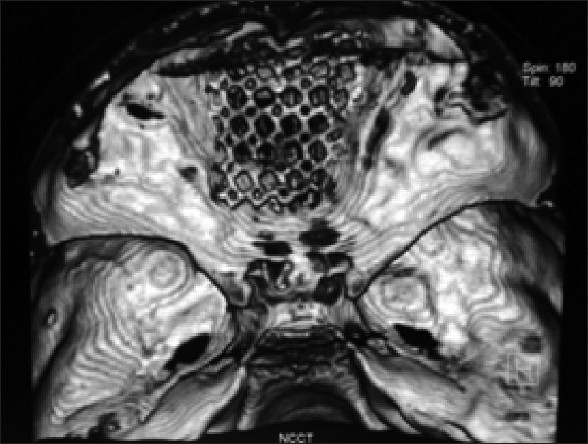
Postoperative CT head with 3D reconstruction showing reconstruction of anterior cranial floor with the titanium mesh
